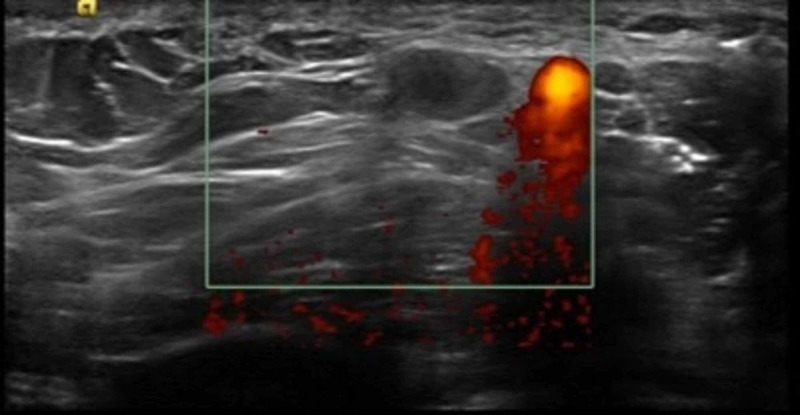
Ultrasonographic image demonstrating hypoechoic lymph nodes without blood flow, adjacent to the axillary vein.
